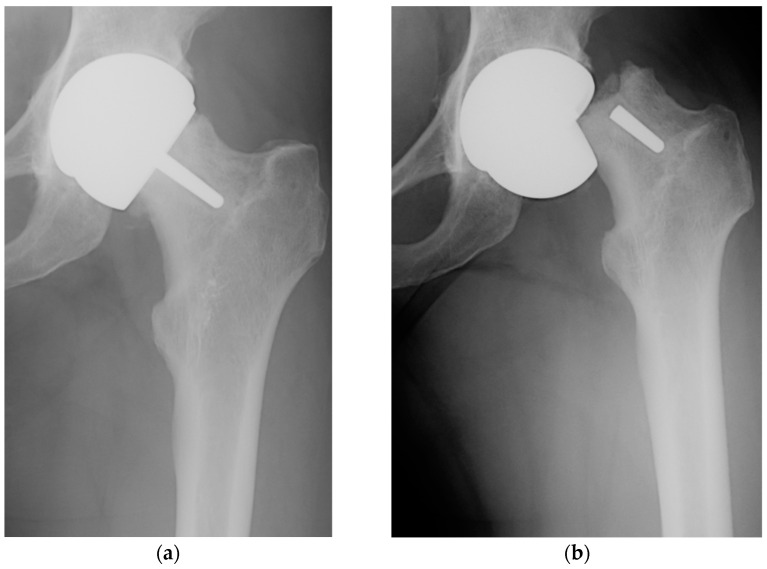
Anteroposterior radiographs demonstrating the Birmingham Hip Resurfacing system in situ (a) with femoral component loosening and (b) femoral neck fracture after one month.
